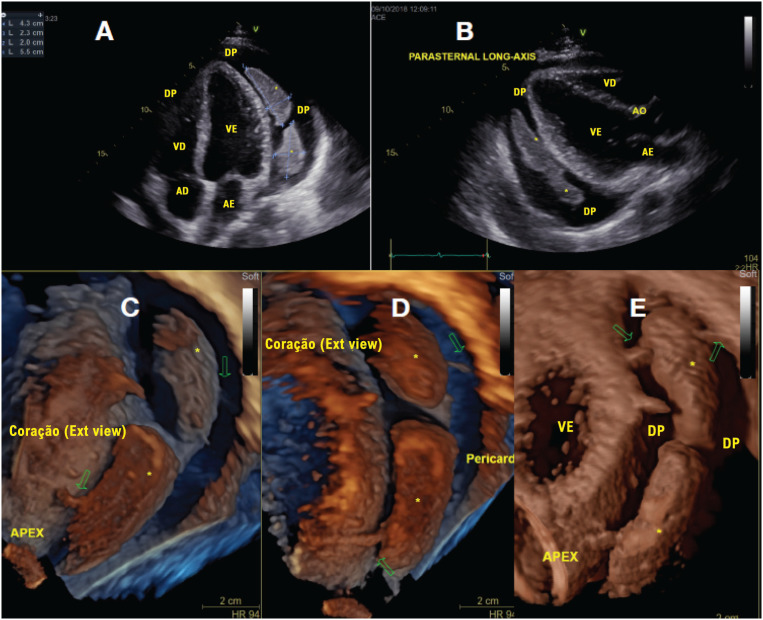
Ecocardiograma transtorácico (ETT) demonstrando volumoso derrame pericárdico com massas intrapericárdicas (*). Ao ECO2D são bem visualizadas duas massas discoides medindo 5.5×2.0cm e 4.3×2.3cm, evidenciáveis a partir das projeções apical 4 câmaras (A) e paraesternal (B) em uma posição póstero-lateral ao coração, em meio ao grande derrame pericárdico. Imagens renderizadas de ECO3D foram obtidas a partir do pós-processamento de “datasets” adquiridos por ETT3D. Podemos evidenciar em C, D e E, em cortes 3D oblíquos, os detalhes morfológicos das massas, com traves fibrinoides que as aderem ao pericárdio parietal e visceral (setas), flutuando dentro do líquido pericárdico e sem invasão de tecidos circunjacentes. VE: ventrículo esquerdo; VD: ventrículo direito; AE: átrio esquerdo; AD: átrio direito; DP: derrame pericárdico.
